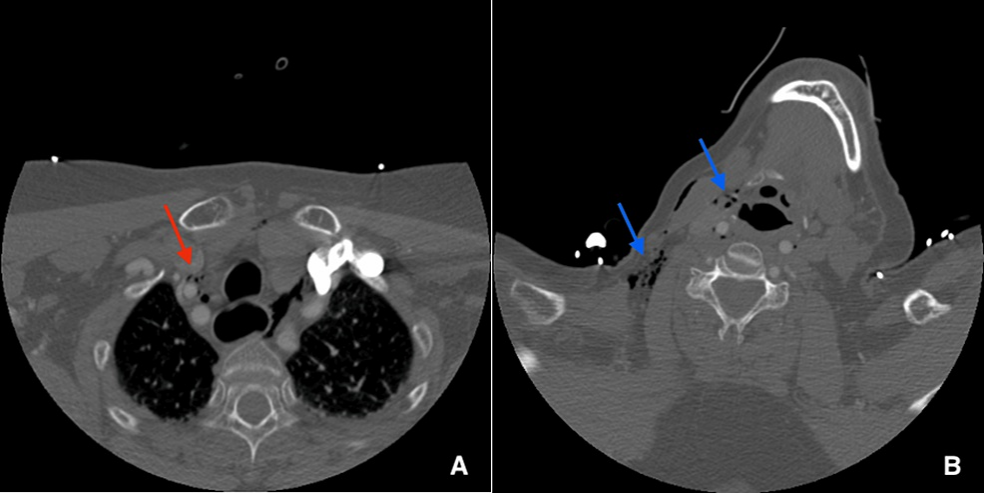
(A) CT chest displaying pneumomediastinum (red arrow); (B) CT neck with subcutaneous emphysema (blue arrows).
